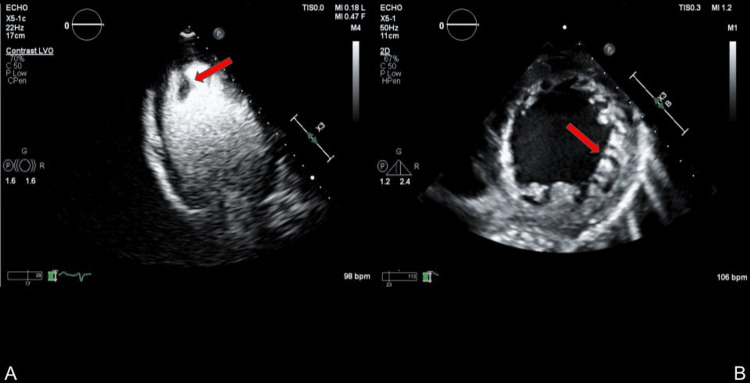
Transthoracic echocardiogram with contrast (A) A 2 cm mobile thrombus at the apex of the left ventricle is visualized. (B) A parasternal short axis view reveals prominent apical trabeculations and deep recesses, consistent with a diagnosis of left ventricular noncompaction (LVNC).
